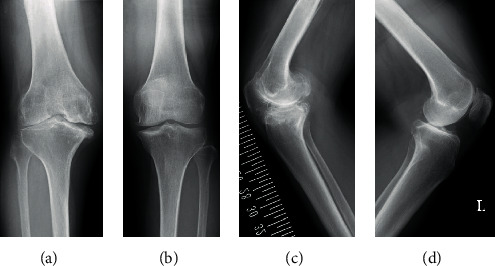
Radiography shows grade 4 osteoarthritis of the right knee joint, according to the Kellgren-Lawrence scoring system, with a pagoda-type tibial plateau. (Right (a) and left (b) anterolateral view and right (c) and left (d) lateral view).
